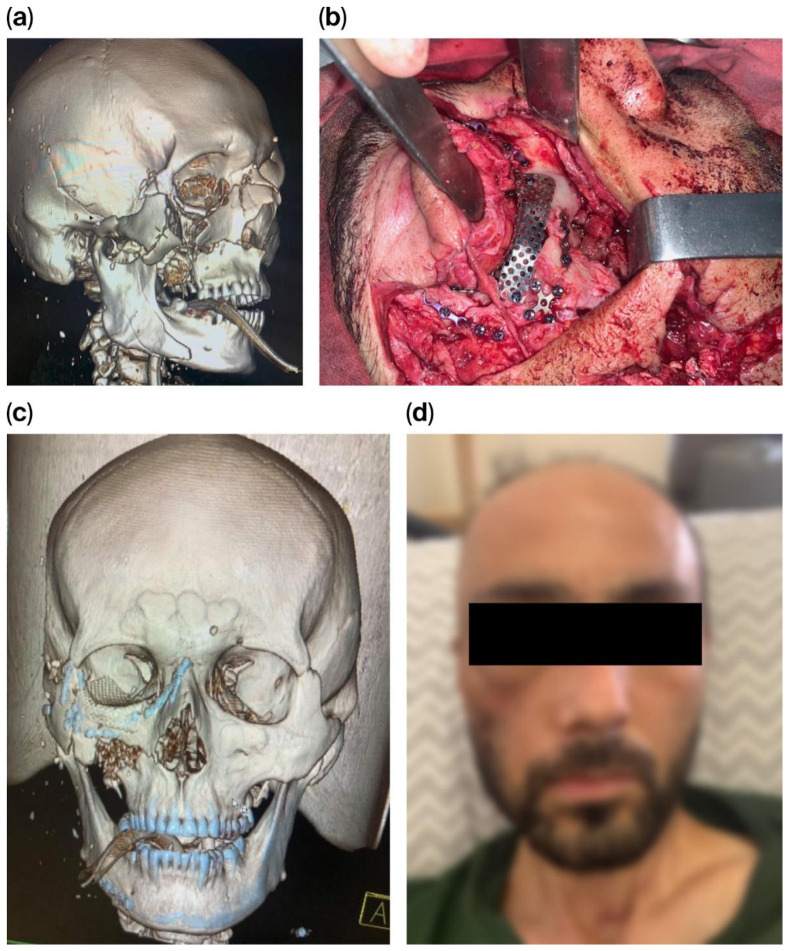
Drone-blast midfacial injury: (a) 3D CT scan demonstrates a right-site severely comminuted midface fracture; (b) early definitive osteosynthesis and blow-out reconstruction using titanium mesh and plates; (c) postoperative 3D CT scan; (d) long-term (5-year follow-up) postoperative result demonstrating soft tissue thinning over titanium mesh and plates in the right zygomatic region.
